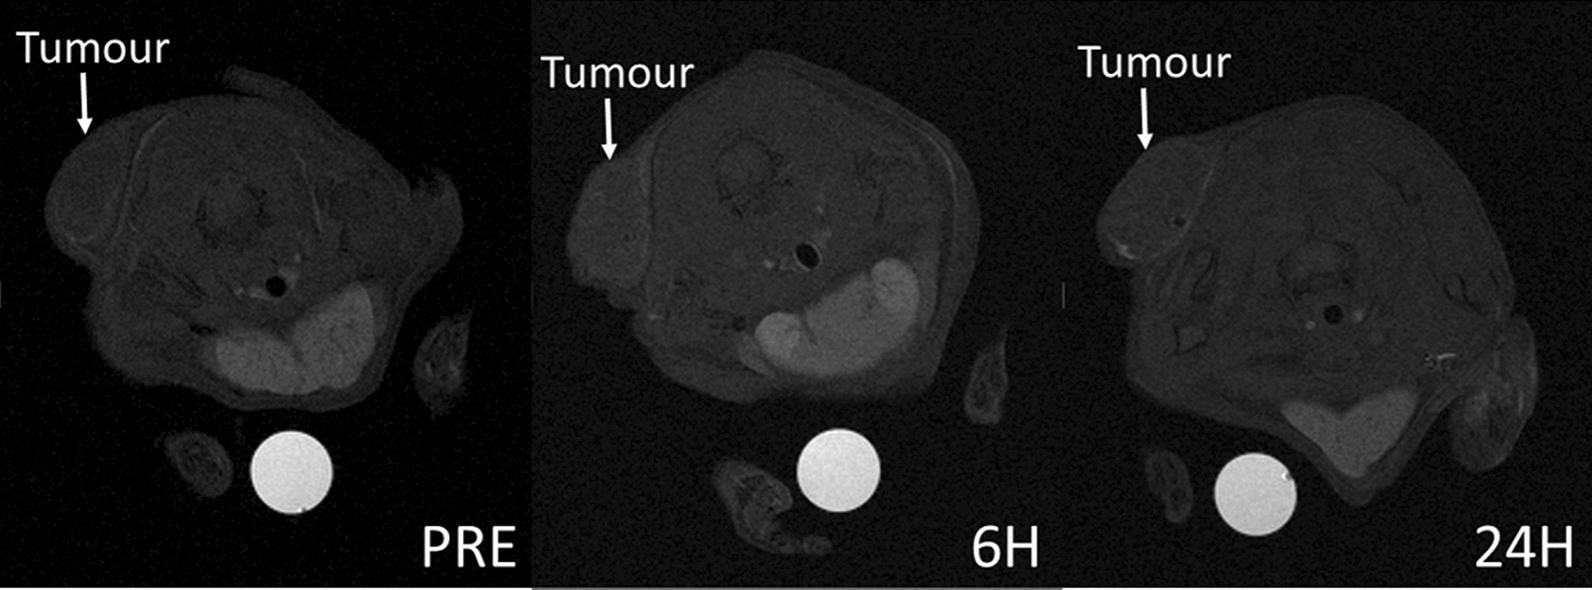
T_1_-weighted spin-echo MR images (7 T) acquired before (PRE), 6 and 24 h after contrast administration
